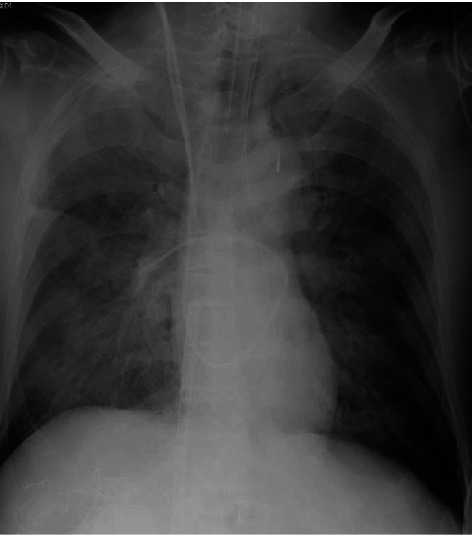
Chest roentgenogram. Chest X-ray showed a severe pulmonary edema.
